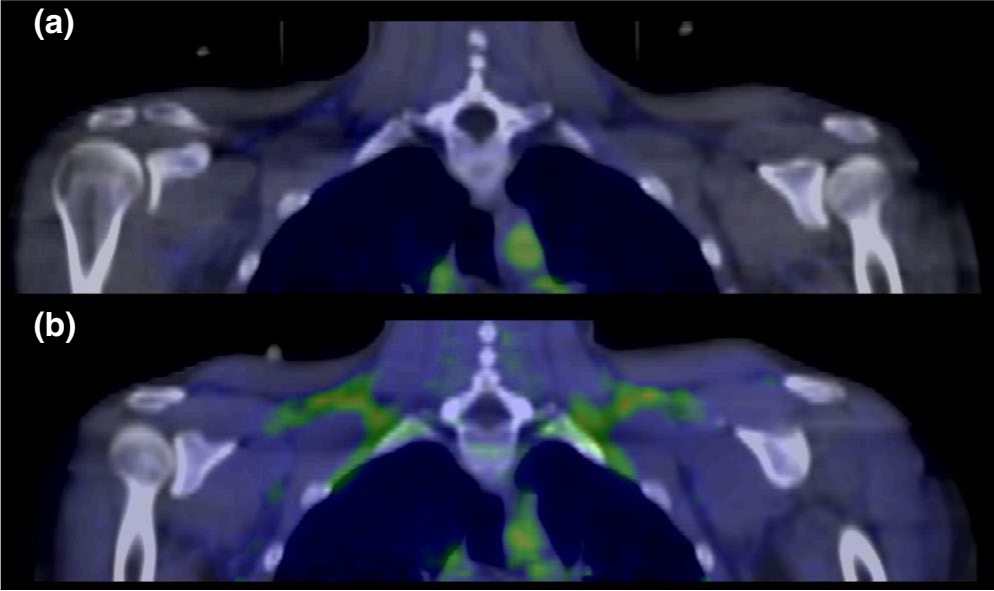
Baseline supraclavicular BAT perfusion in room temperature during fasting (a) and elevated BAT perfusion during cold exposure in the same subject (b). Highest tracer activity is marked with green in the PET/CT fusion images.
